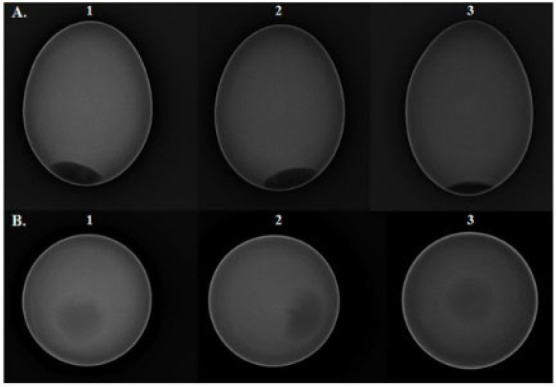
Different quality egg X-ray imaging (reproduced from ref. [128]). (A) median and (B) axial plane images. (1) special organic eggs, (2) general organic eggs, and (3) conventional eggs.
